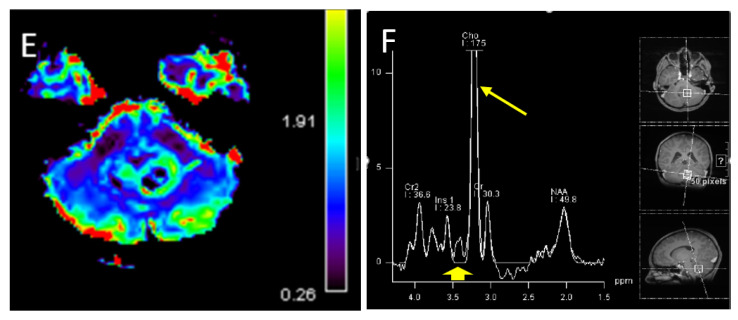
A 7-year-old boy with medulloblastoma. Axial T2 (A), sagittal post-contrast T1 (B), images demonstrate a mildly heterogeneous midline posterior fossa mass. DWI (C) and ADC (D) imaging demonstrates diffusion restriction of the lesion relative to the adjacent cerebellar parenchyma, typical of these highly cellular neoplasms. Perfusion imaging (E) demonstrates increased relative cerebral blood volume within the lesion and MRS (F) demonstrates a high choline peak (long arrow) as well as a small taurine peak (short arrow).
